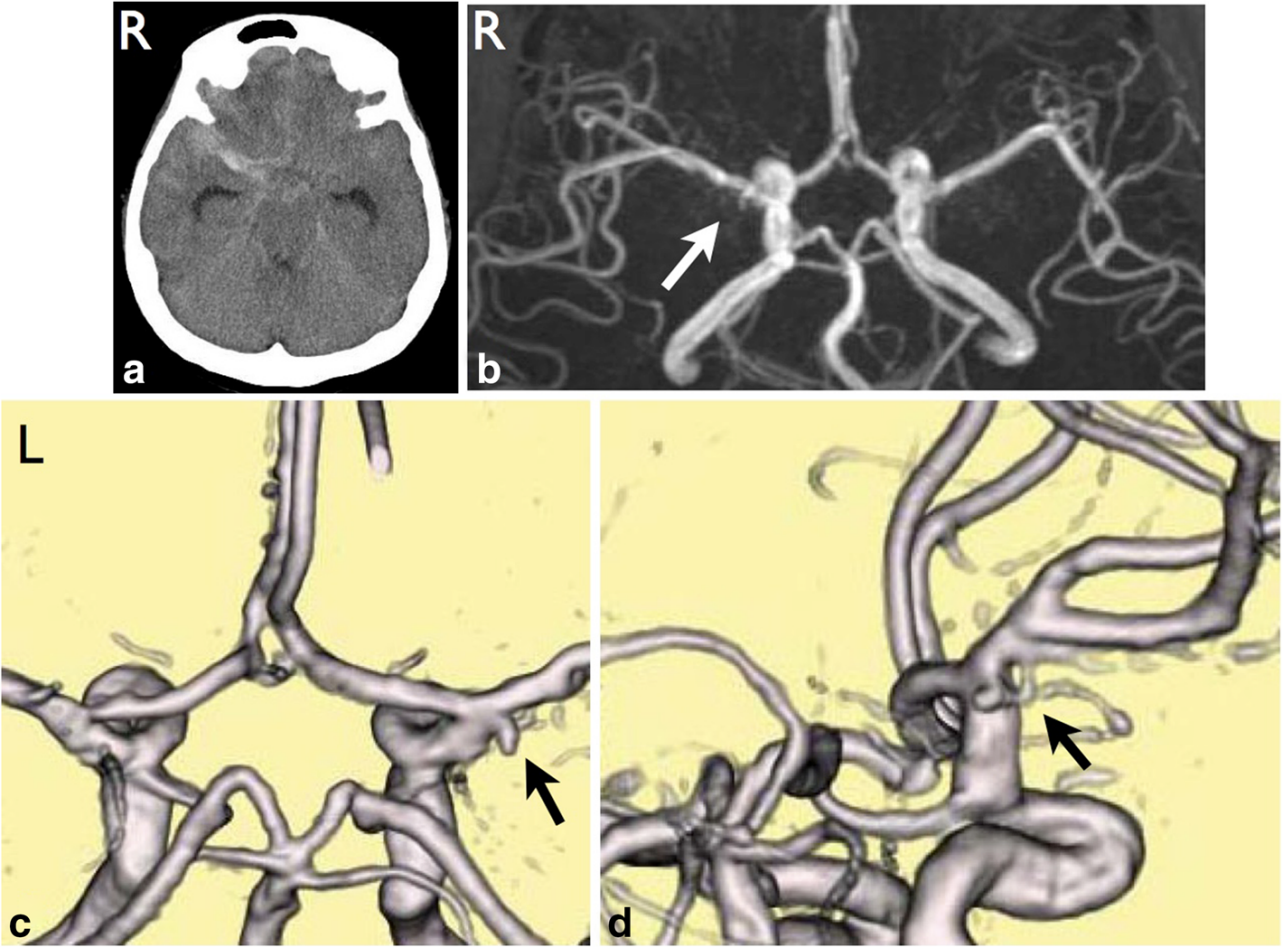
Pre-operative images. (a) Plain computed tomography shows subarachnoid hemorrhage in the right Sylvian fissure. (b) Maximum intensity projection image of magnetic resonance angiography suggests the presence of a ruptured aneurysm at the right proximal M1 portion (arrow). (c) Volume rendering image of magnetic resonance angiography shows the aneurysm arising at the fenestration of M1 (arrow). (d) Different angle of the volume rendering image shows the anatomical relationship between the fenestration and the aneurysm (arrow). R, Right side; L, Left side.
